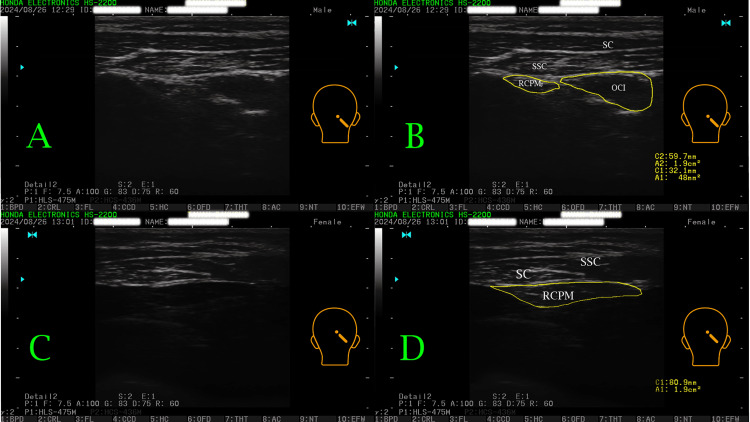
Sonogram of RCPM, RCPMJ, and OCI (A) OCI and RCPMJ sonograms without area measurements. (B) OCI and RCPMJ sonograms with yellow borders outlining the area measurements, including measurement values. (C) RCPM sonogram without area measurement. (D) RCPM sonogram with a yellow border outlining the area measurement, including measurement values. RCPM: rectus capitis posterior minor; RCPMJ: rectus capitis posterior major; OCI: obliquus capitis inferior
